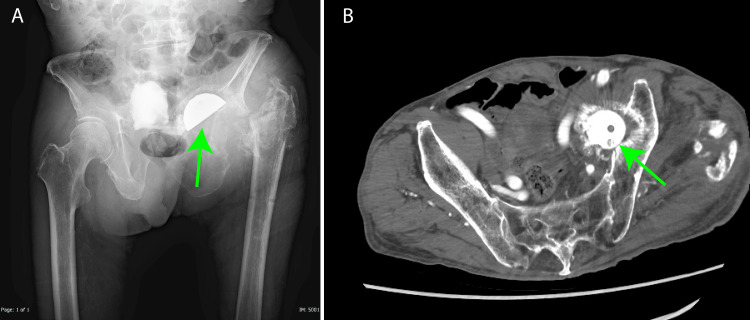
Preoperative images A) The joint cup is displaced medially beyond the acetabular cap (green arrow), as shown in the X-ray, and the left femoral bone head was already resected B) Enhanced computed tomography image revealed a cup located in the pelvis (green arrow)
